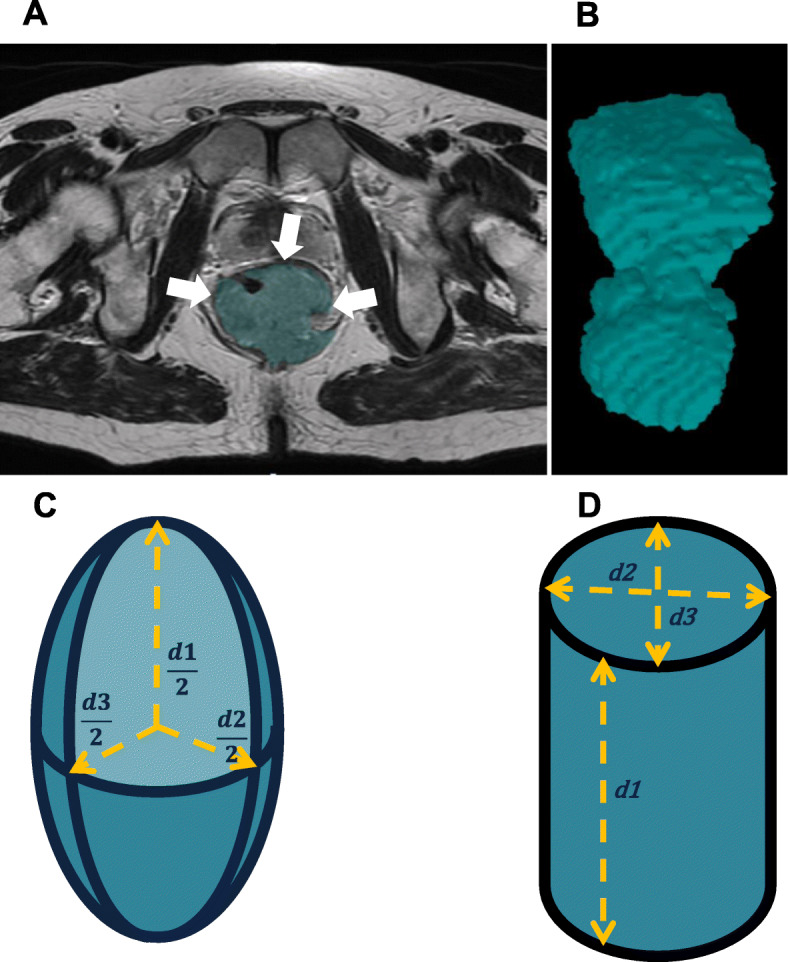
Method of quantification of tumour volume: a pelvic axial section of MR images depicting an anal canal tumour contoured in blue (arrows); b sequential contours rendered to create 3D representation of the tumour, demonstrating its irregular and complex shape; and c estimation of volume using orthogonal tumour size measurements under the assumption that the tumour volume approximates an ellipsoid. d estimation of volume using orthogonal tumour size measurements under the assumption that the tumour volume approximates an elliptical cylinder. Figures (a) and (b) were created using World-Match (inhouse written software). Figures (c) and (d) were created using Microsoft Office Powerpoint
